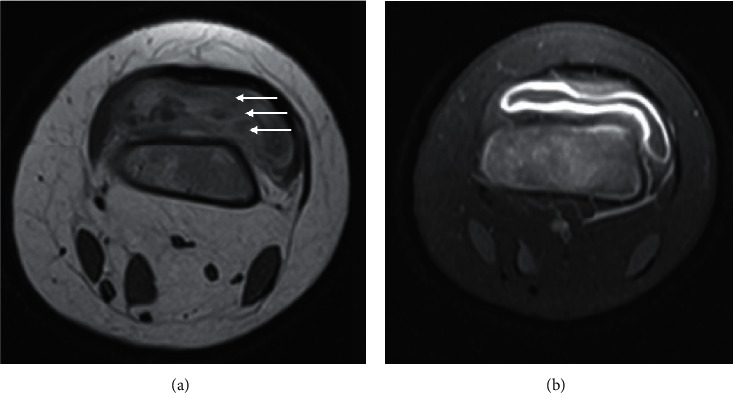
Axial T2 (a) and T1FS postcontrast (b) MRI of the knee in a 6-year-old boy. Lamellated synovial thickening (a) is specific for septic arthritis.
